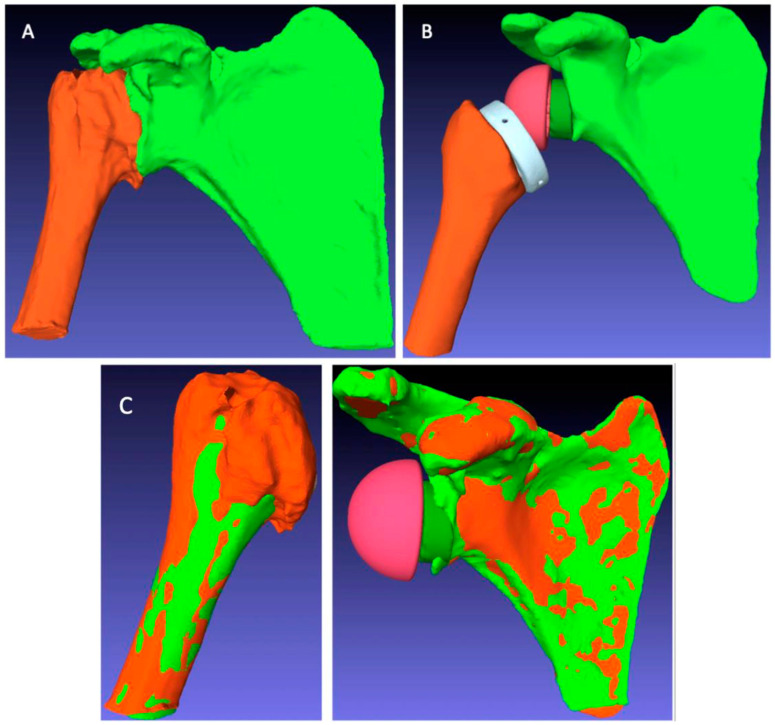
Image processing steps for postoperative arm change position (ACP) measurement: (A) automatic segmentation to extract the humeral and scapular bones from the postoperative CT scan; (B) manual segmentation to extract the bones and implants from the postoperative CT scan; (C) registration overlay of the preoperative (green) and postoperative (orange) structures to measure the ACP corresponding to the postoperative situation.
